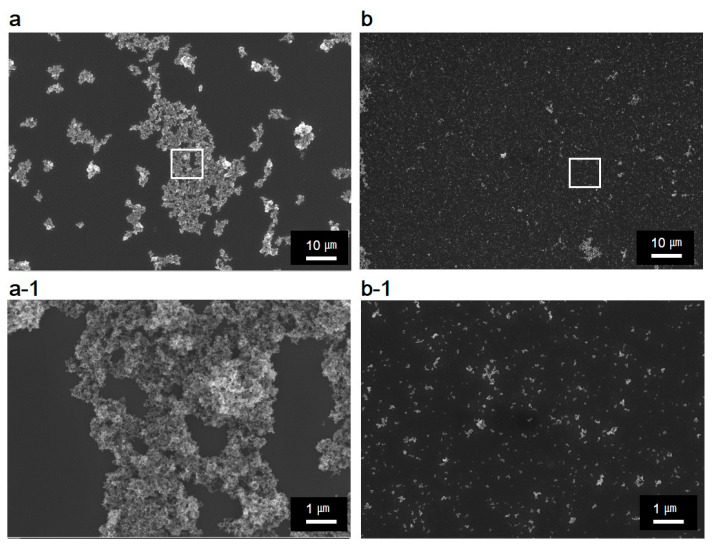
SEM images before (a) and after (b) ultrasonic dispersion. (a-1) and (b-1) represent the magnified images of the regions marked in white boxes in (a) and (b), respectively.
